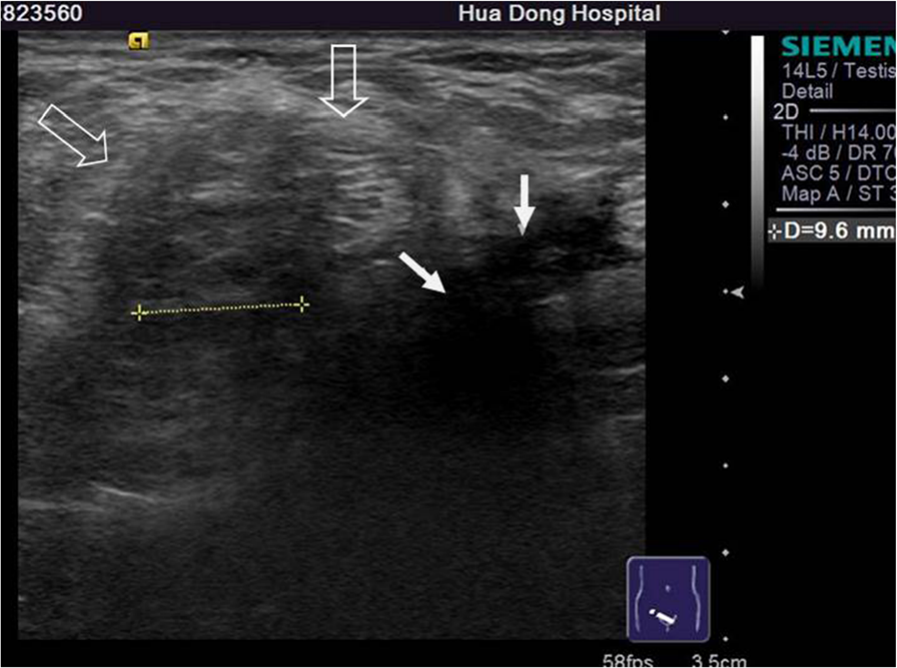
The recurrent hernia and the shrinking mesh showed in the ultrasonography. Hollow arrows referred to the recurrent hernia and solid arrows referred to the shrinking mesh. The measurement of straight line referred to the diameter of the perito neal defect of the recurrent hernia
